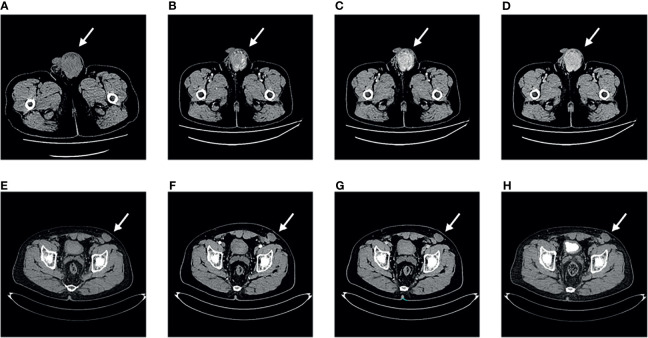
Computed tomography findings. Pelvic computed tomography of primary tumour (A–D). (A) Plain scan. (B) Arterial phase. (C) Venous phase. (D) Excretion phase. Pelvic computed tomography of recurrent tumour (E–H). (E) Plain scan. (F) Arterial phase. (G) Venous phase. (H) Excretion phase.
